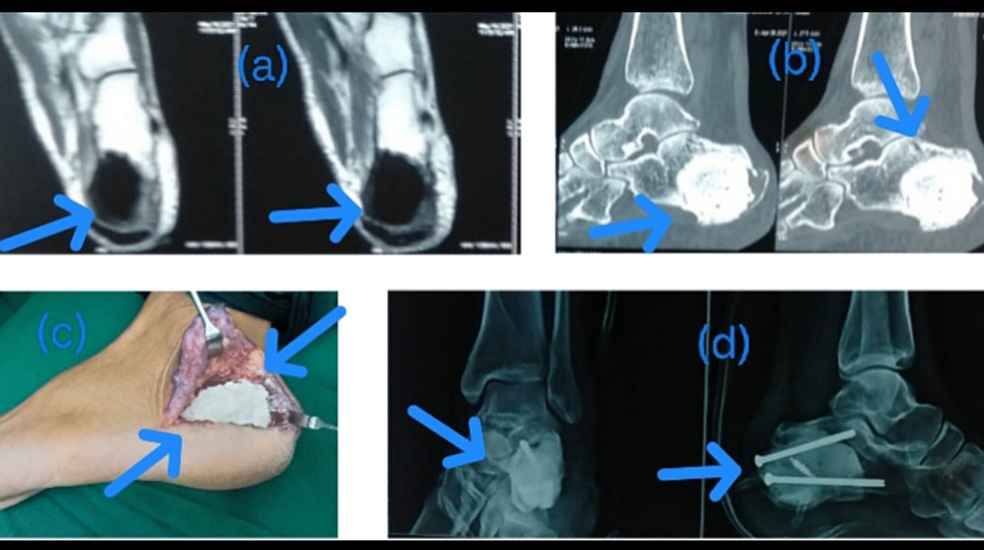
Calcaneum giant cell tumor (GCT) A 24-year-old male patient of GCT presented with a complaint of heel pain. After MRI (a) and CT scan (b) evaluation, and confirmation, it was treated with curettage (c) and cementing (d).
